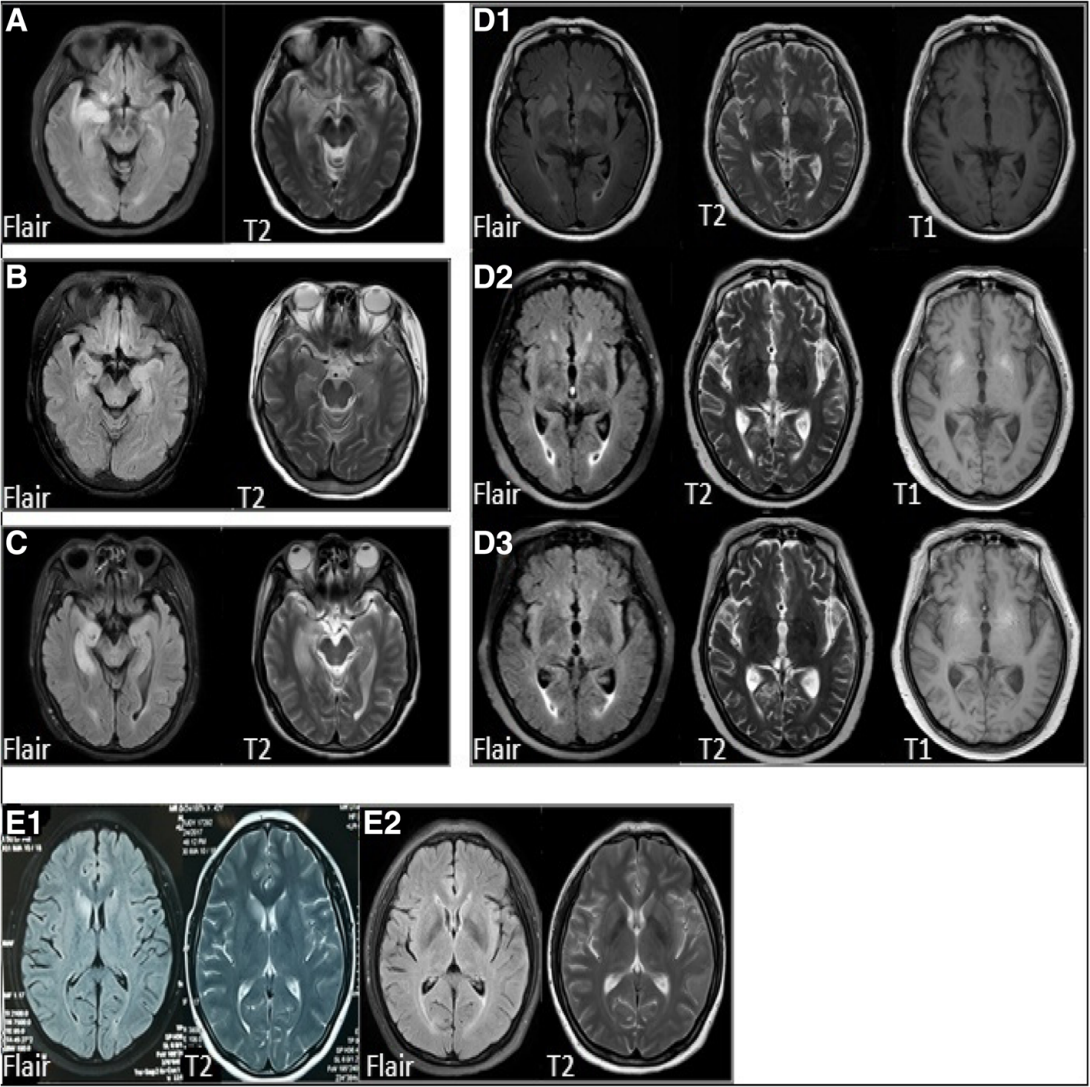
MRI images of five patients with LGI1 antibody encephalitis. a FLAIR sequences and high T2 signal changes in the right insula in case 1; b FLAIR sequences and high T2 signal changes in the bilateral hippocampus in case 3; c FLAIR sequences and high T2 signal changes in the right hippocampus in case 5; D1 Abnormal signals in the bilateral caudate nucleus and putamen in case 4 at admission; MRI follow-up after 1 month (D2) and 2 months (D3) showed that the lesion signal intensity changed to a high T1 signal; (E1) Abnormal signals in bilateral caudate nucleus in case 7 at admission; E2 MRI of case 7 after 5 months. Abbreviations: MRI, magnetic resonance imaging; FLAIR, fluid-attenuated inversion recovery
